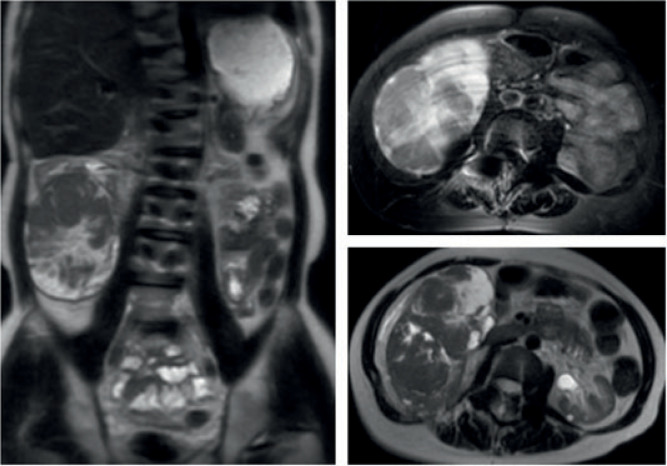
MRI imaging of abdomen without IV contrast: Multiplanar T1- and T2-weighted images of the abdomen were obtained without IV contrast. A large and complex mass is seen arising from the right kidney lower pole measuring 11.6 × 8.3 × 12.7 cm.
